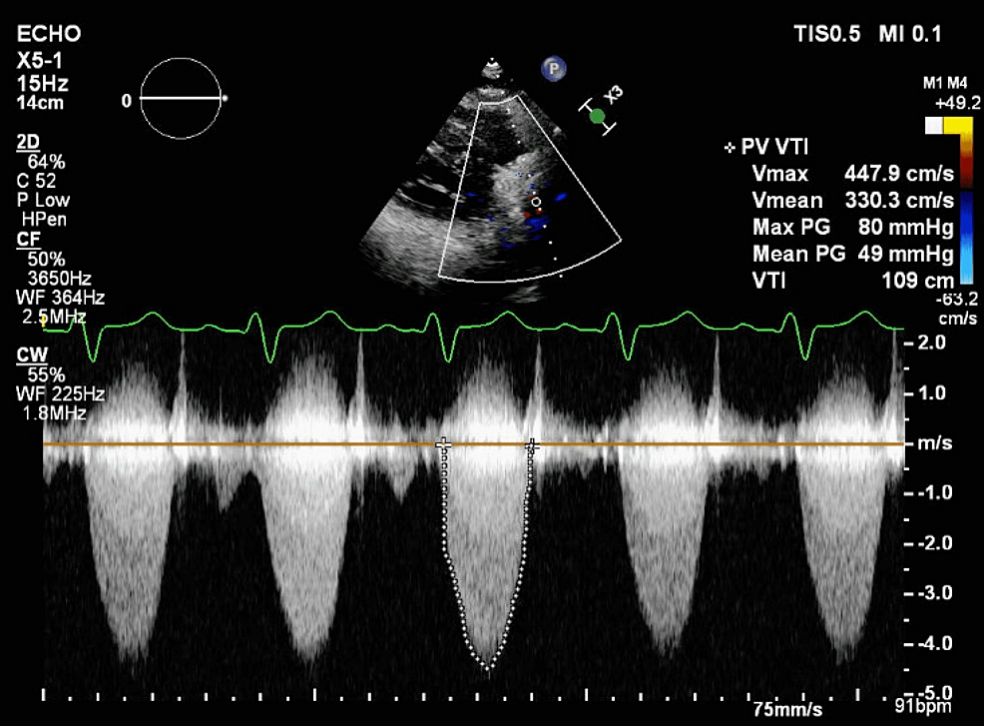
TTE showing significant severe obstruction across Melody valve TTE: transthoracic echocardiogram
